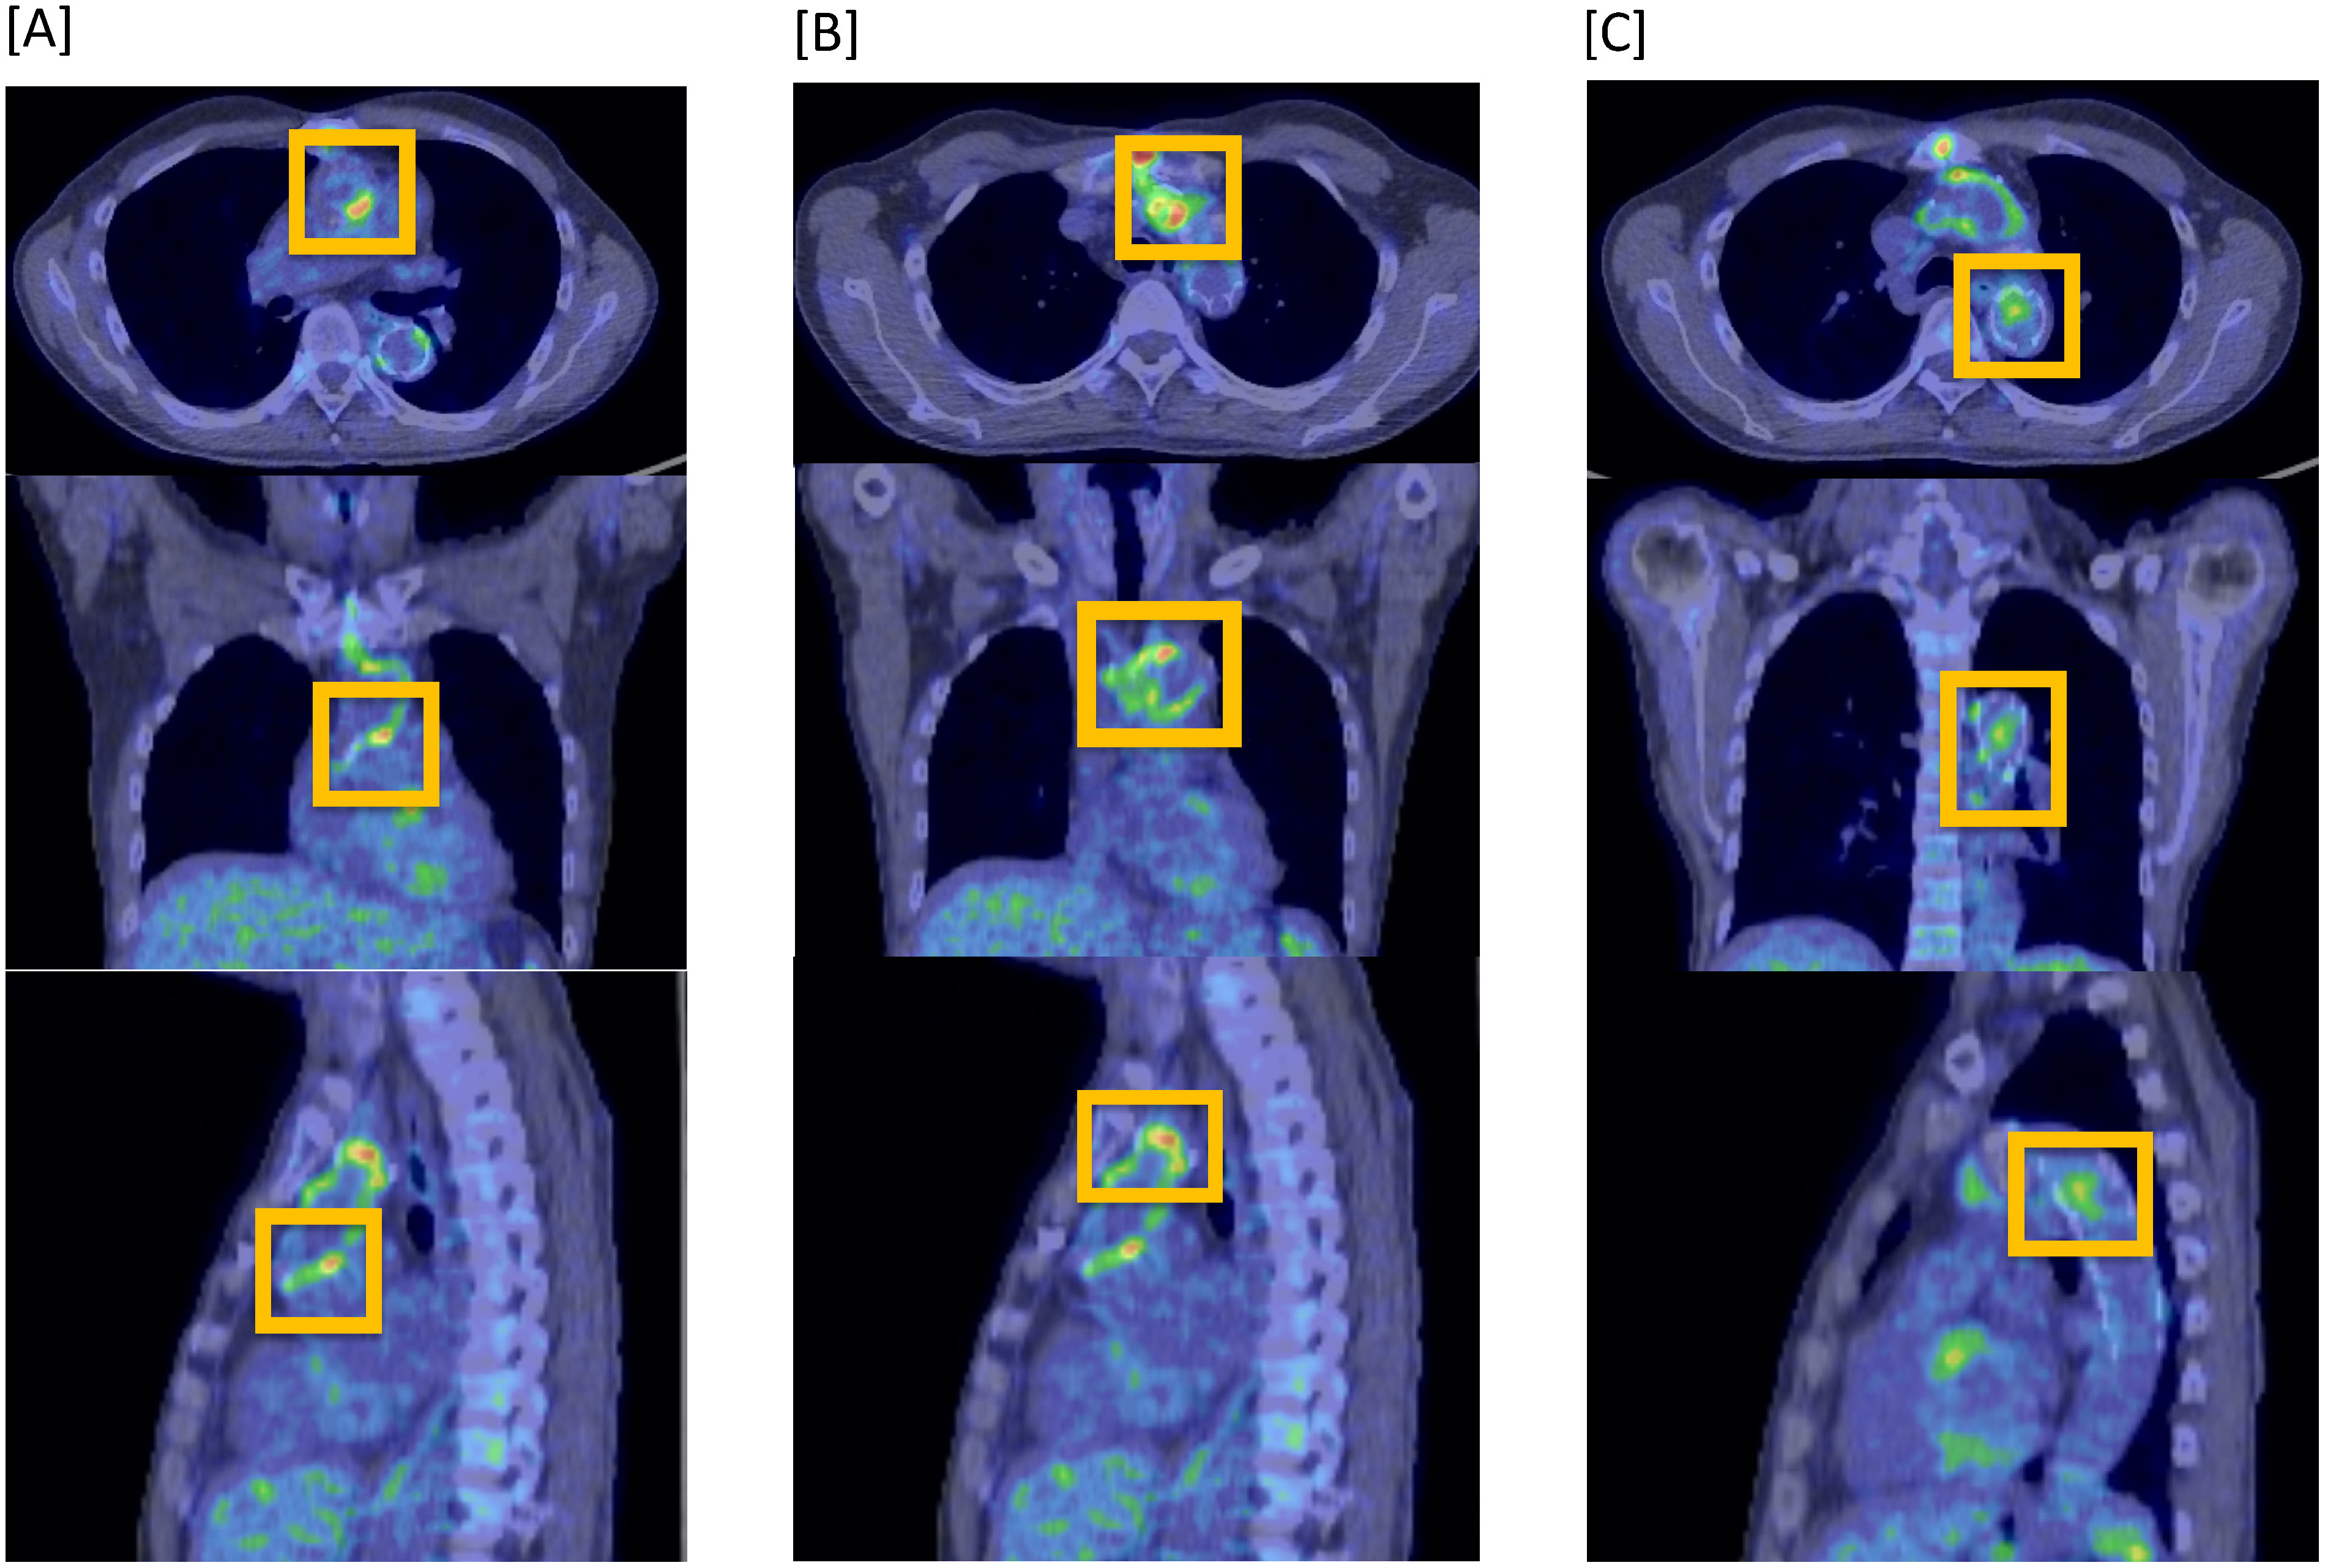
Fig. 2 Fusional FDG PET/CT images. From top to bottom, the axial view, coronal view, and sagittal view are listed. Orange rectangles: high uptake area on FDG PET/CT.
